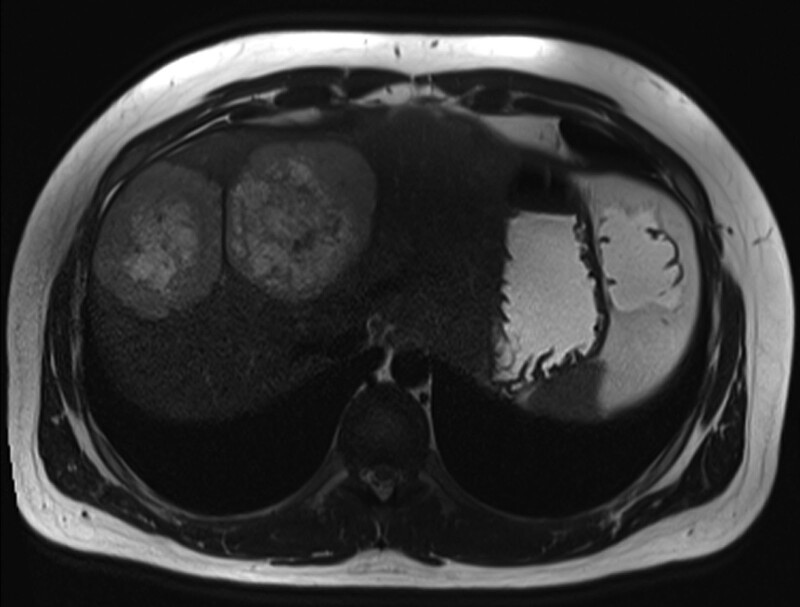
T2-weighted axial haste magnetic resonance imaging sequence demonstrates 2 right lobe liver lesions with target sign. Periphery of lesions (viable tumor) is relatively hypointense compared with center (liquefactive necrosis).
